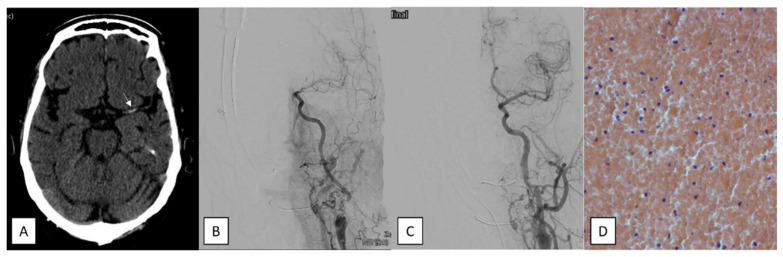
A 75-year-old female that was admitted with acute stroke with a NIHSS score of 11 points due to tandem occlusion (left proximal internal carotid artery stenosis and M1 occlusion). (A) A non-contrast head CT showing the left-sided hyperdense vessel sign in the middle cerebral artery (arrow). (B) Digital subtraction angiography confirmed the tandem occlusion. (C) Control angiogram showed complete recanalization of the middle cerebral artery with a TICI score of 3 after the first pass with stent retriever, as well as by showing concominant extracranial angioplasty and stenting of the proximal internal carotid artery lesion. (D) An obvious HE20x accumulation of erythrocytes with little inflammatory components and no fibrin and platelets.
